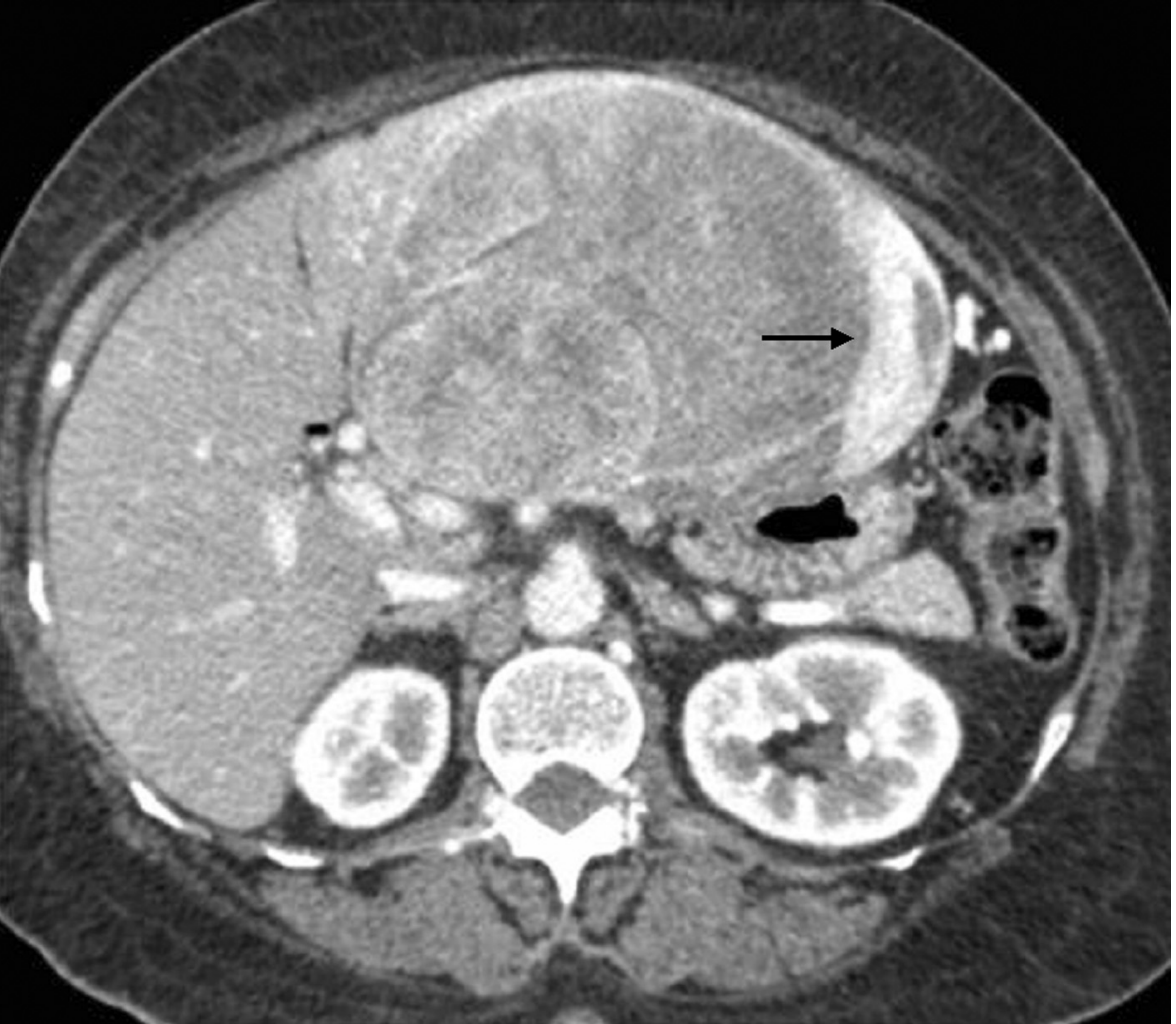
Portal-stage contrast-enhanced helical CT of the upper abdomen. Impregnation by the contrast agent shows a large, solid, exofitic lesion compromising the left liver lobe. The enhancement is intense and heterogeneous, the dominant portion being in the periphery of the lesion (arrow).
